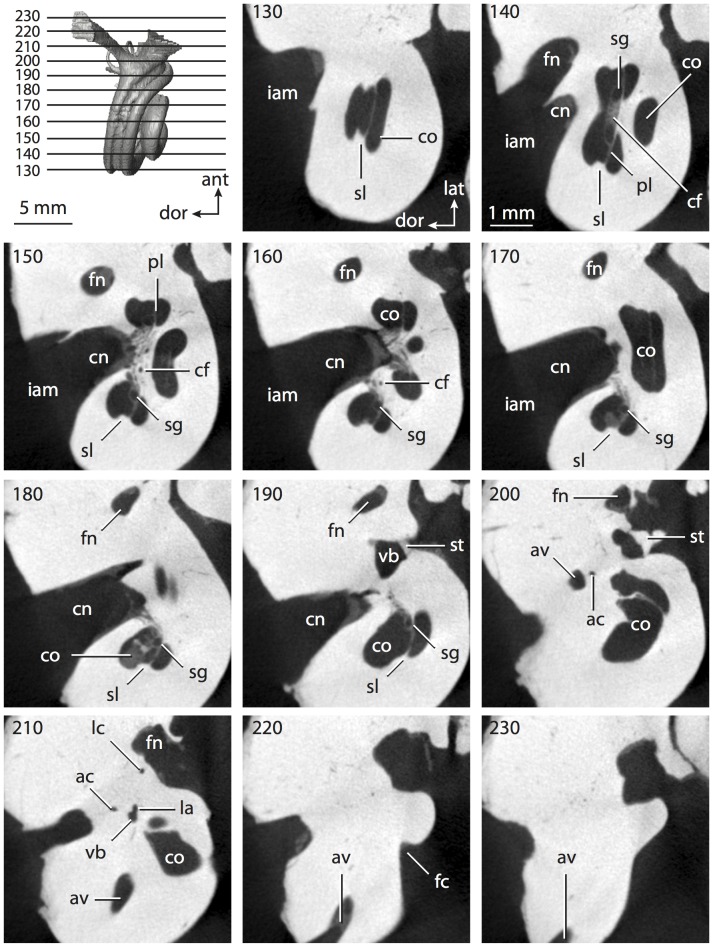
CT slices through ear region of*Tursiops truncatus*. Abbreviations listed at the end of the Materials and Methods section.
